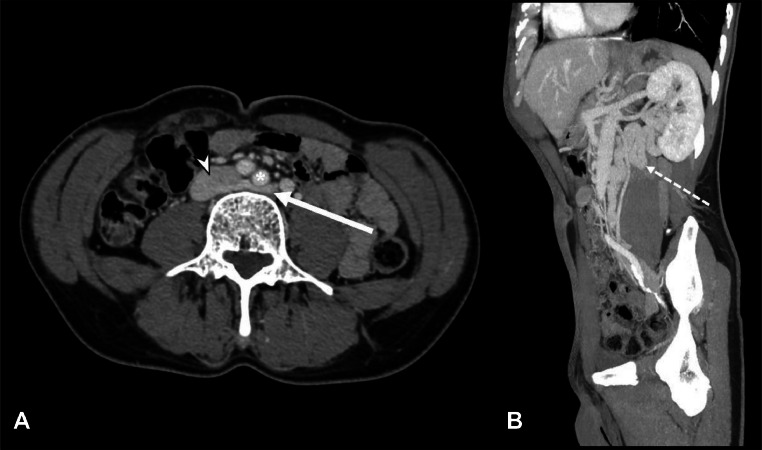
(A,B) Retroaortic left venal rein compression. Axial CE-CT in venous phase (A) shows the retroaortic renal vein (arrow) compressed by the left iliac artery (asterisk), before flowing into the inferior vena cava (arrowhead). Furthermore, a parasagittal section obtained by MIP reconstruction (B) shows considerable ectasia of the renal vein tract upstream of the compression (dotted arrow in B).
